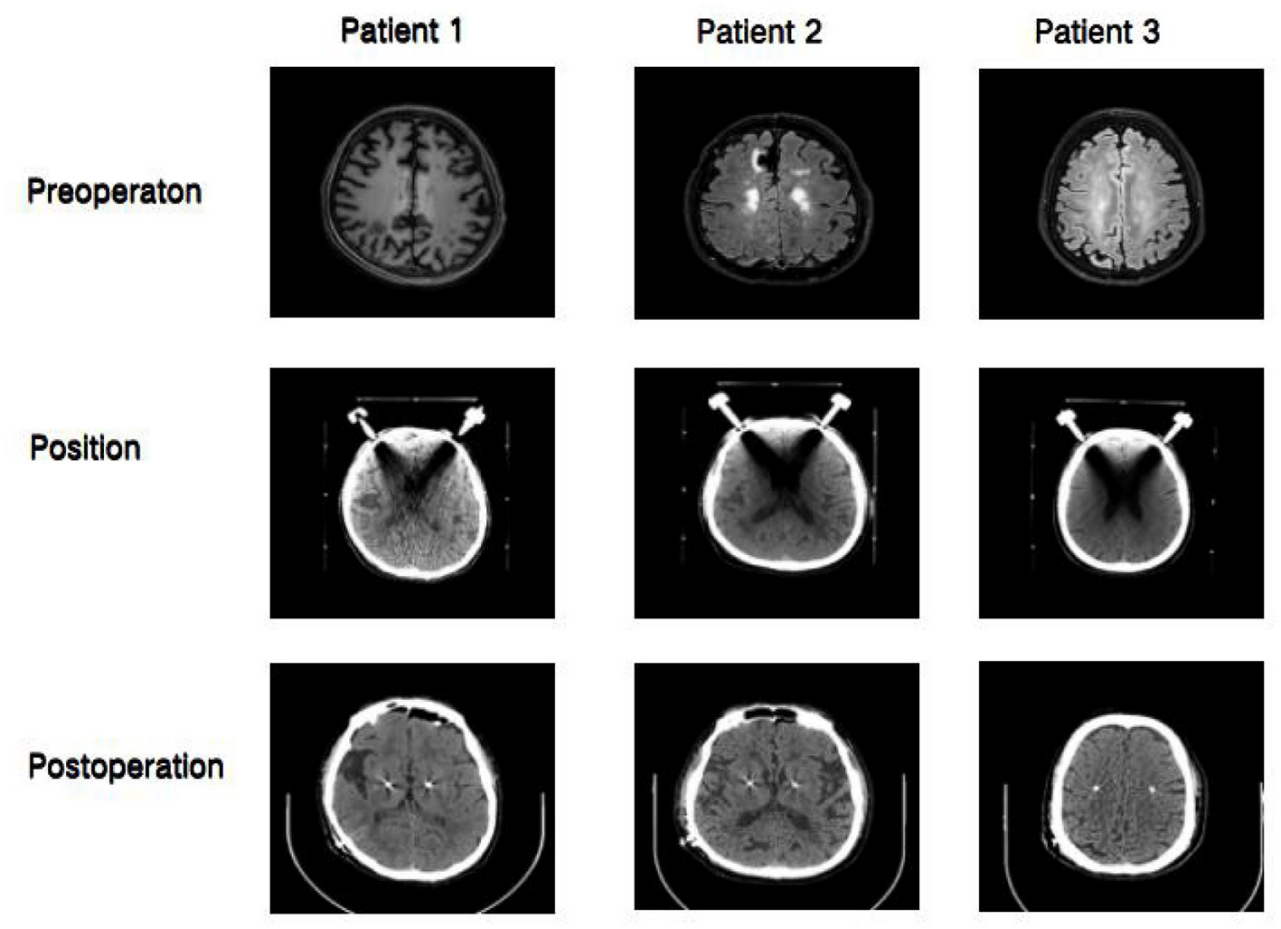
Preoperative, electrode localization, and postoperative brain CT. Three patients underwent brain CT before operation, electrode positioning and after operation. The patient was admitted to the hospital for preoperative examination of the head CT (Preoperation); Before surgery upper head frame positioning CT (Positon); After surgery to determine the electrode position CT (Postoperation;).
